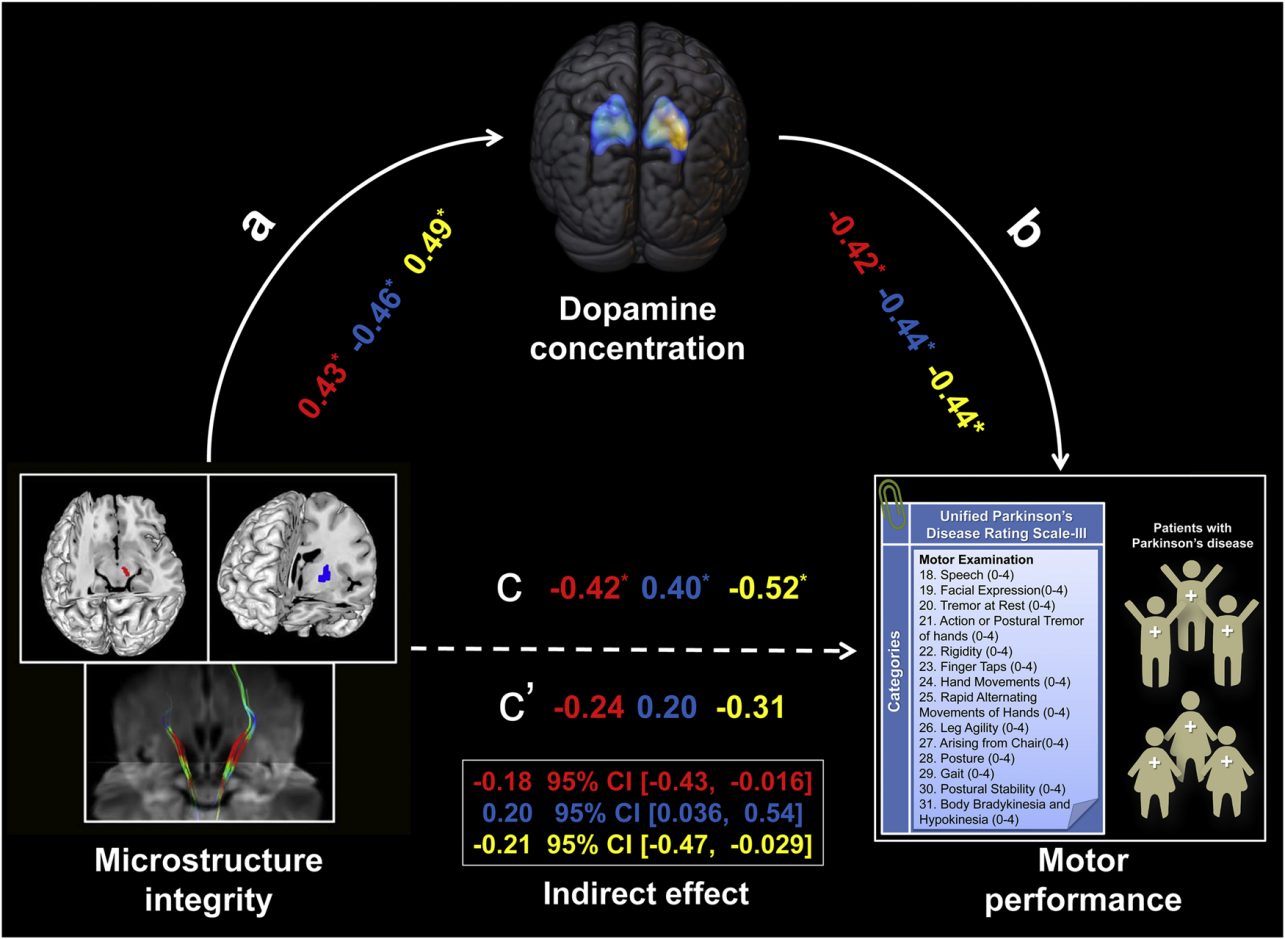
The mediation effect of dopaminergic function on the more affected side of PD. Dopamine concentration (putaminal SUVR) significantly mediated the relationship between microstructure integrity (FA in SN, red; MD in putamen, blue; FA of nigrostriatal projection, yellow) and motor performance (UPDRS-III scores). a, b, c, and c’ indicate path coefficients; * indicates significant difference (*P* < 0.05). Abbreviations: PD Parkinson’s disease, SUVR standard uptake value ratio, FA fractional anisotropy, MD mean diffusivity, UPDRS-III Unified Parkinson’s Disease Rating Scale III, CI confidence interval.
